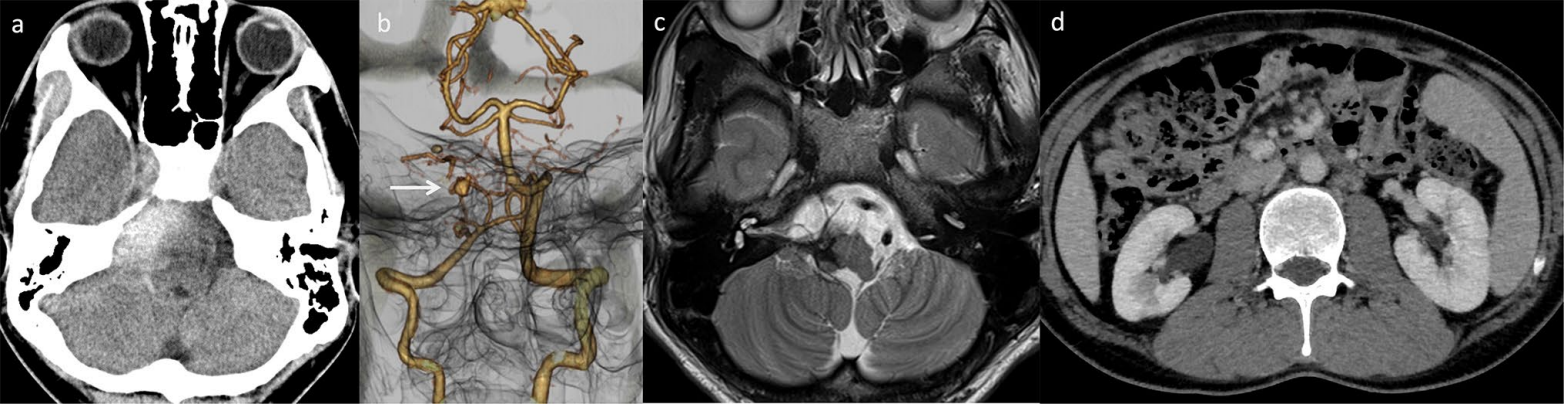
A 26-year-old, male patient, the sibling of the patient mentioned above (Fig. 12), with deficiency of adenosine deaminase type 2 (DADA2). He had a history of recurrent diplopia, sensory numbness, and right-sided hearing impairment since the age of approximately 10 years. His cutaneous symptoms consisted of rash (livedo racemosa) and a history of frostbite. At the age of 22 years, DADA2 caused by defective ADA2 activity in the plasma was diagnosed (this patient has already been reported by Nihira et al. “P2” corresponds to this case) [142]. a Initial head non-contrast computed tomography (CT) revealed a parenchymal hemorrhage on the right side of the pons and a subarachnoid hemorrhage along the prepontine cistern. b Subsequent CT angiography indicated a possible pseudoaneurysm of the right anterior inferior cerebellar artery (AICA) (arrow). c Follow-up T2-weighted imaging demonstrated hemosiderin deposition around the brainstem region, suggesting previous, hemorrhagic events. d Contrast-enhanced CT highlighted impaired renal cortical enhancement and irregularities suggestive of a previous renal infarction. Splenomegaly was also observed
